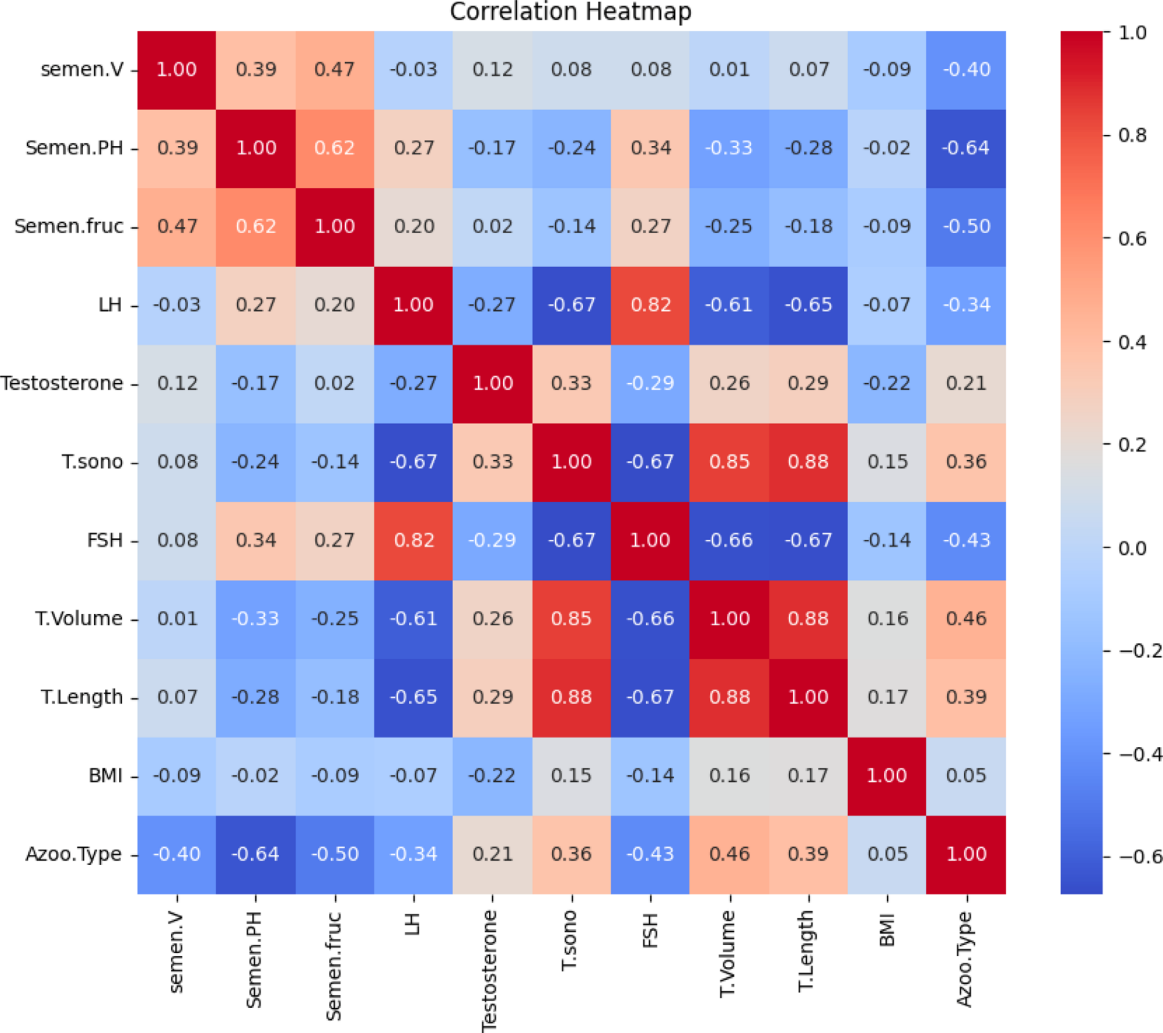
Correlation heat map of variables included in the machine learning‐based modelling; Azoo, Type of azoospermia; BMI, body mass index; FSH, follicle‐stimulating hormone; LH, luteinizing hormone; semen.fruc, semen fructose; semenPH, semen pH; semen. V, semen volume; T. Length, testis length; T.sono, testis longitudinal axis in ultrasonography; T. Volume, testis volume.
